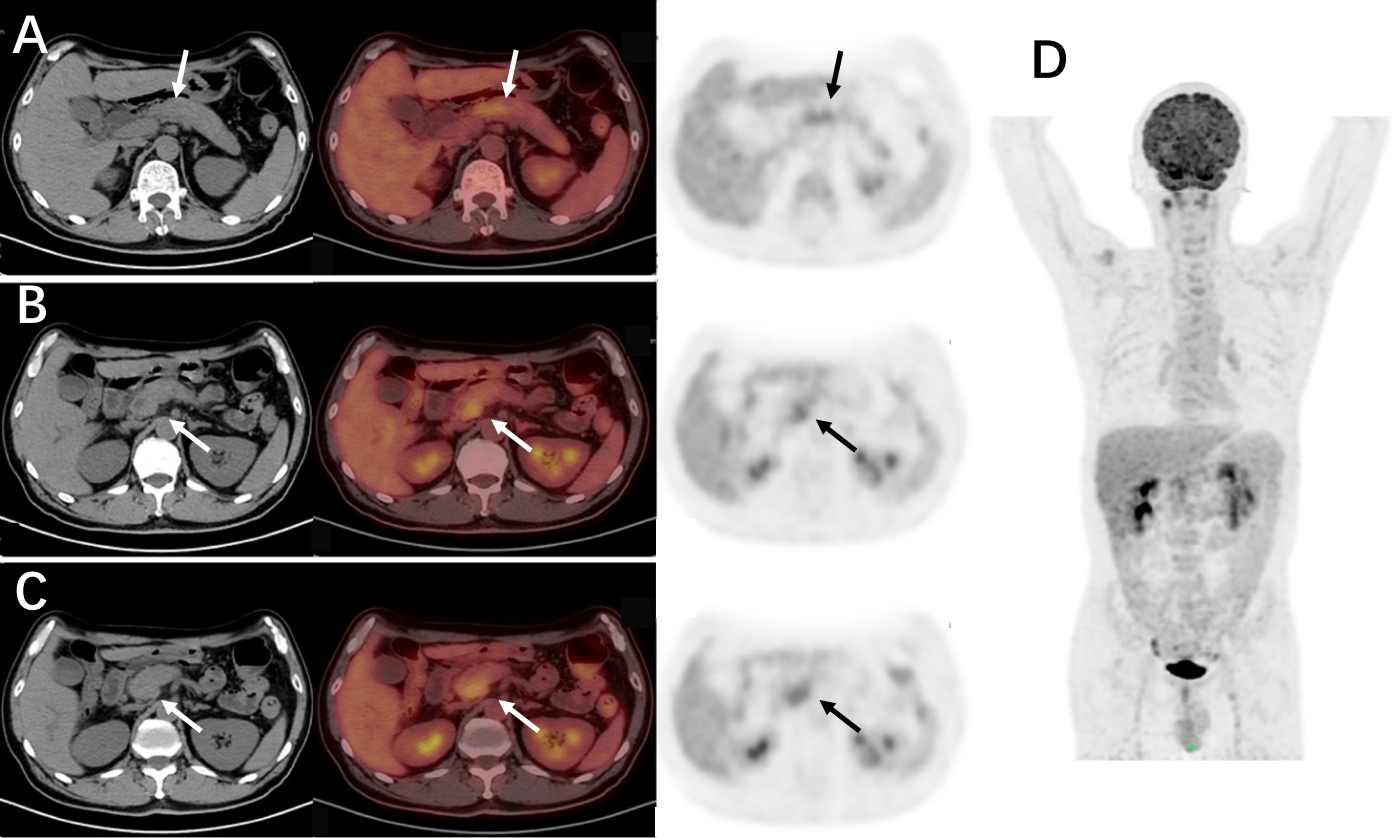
^18^F-FDG PET/CT images of IgG4-RD; the mild increase of FDG uptake in uncinate process and neck of pancreas was difficult to differentiate from pancreatic cancer
